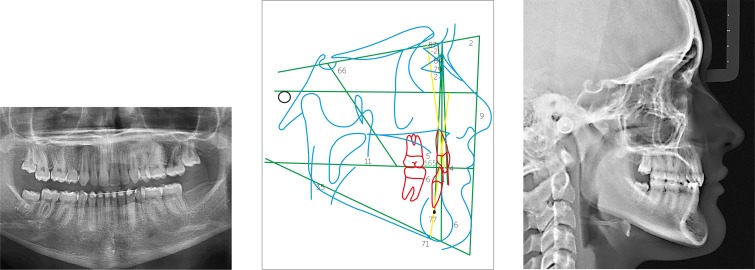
Panoramic radiograph reveals satisfactory root parallelism and total space
